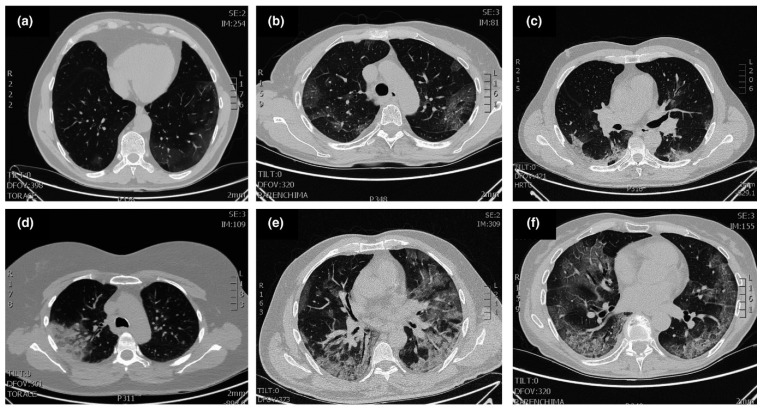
Typical patterns of COVID-19 at CT imaging. (a) Ground glass shadows (early stage), (b) Ground glass opacities, (c) Ground glass nodules and subpleural consolidation, (d) Focal consolidation, (e) Multifocal consolidation, (f) Multifocal consolidation with honeycomb (end stage). Reproduced with permission. Ref. [1] Journal of Internal Medicine © 2022 John Wiley and Sons, Inc.
